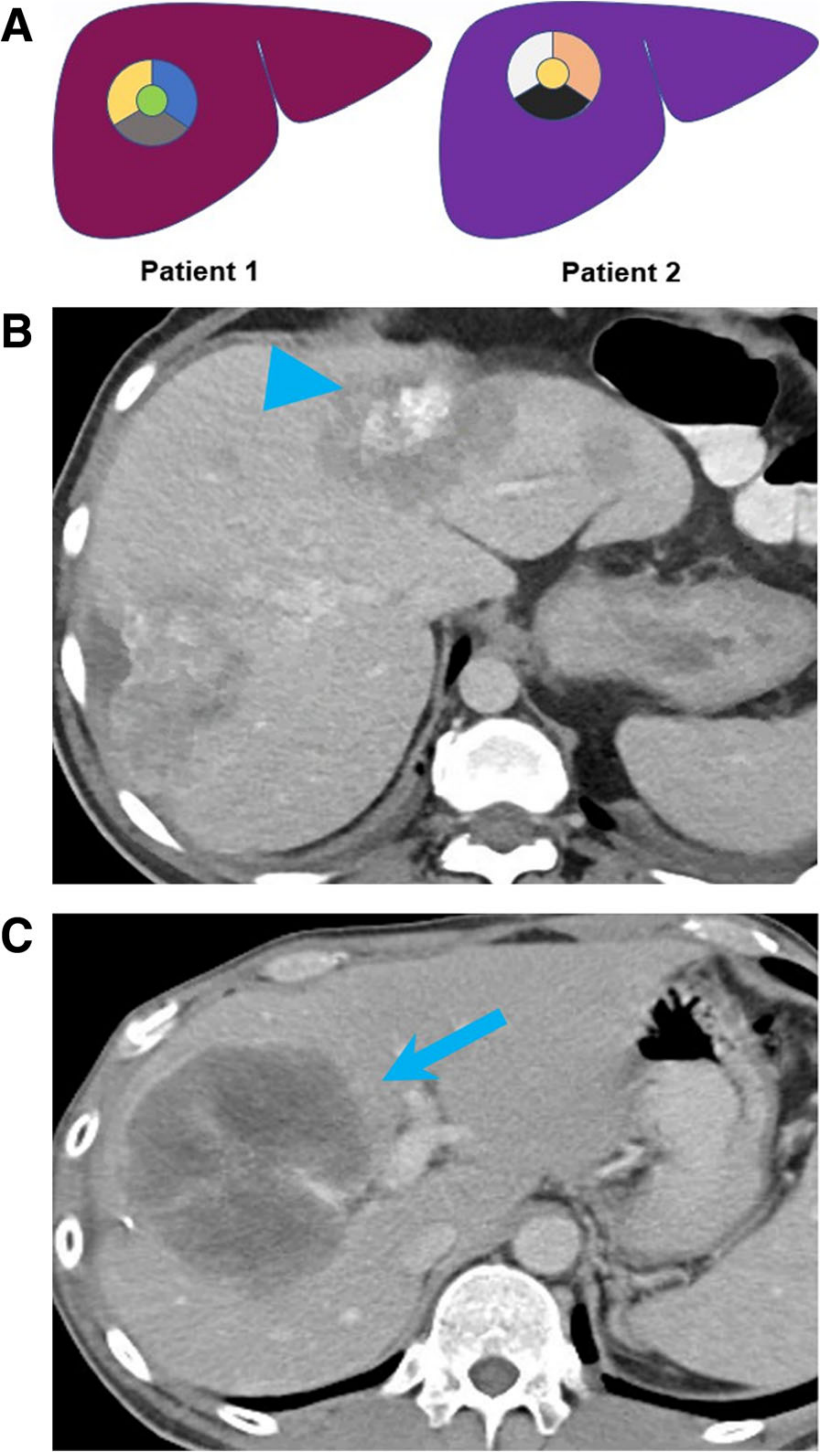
Interpatient heterogeneity. a Image showing mutational heterogeneity among tumors of different patients, where different mutations are depicted in different colors. b, c Axial CT images acquired during portal venous phase in two different patients with pathological diagnosis of metastatic adenocarcinoma of the colon. One patient shows partially calcified metastases (arrowhead) (b); one patient shows hypodense heterogeneously enhancing masses (c)
